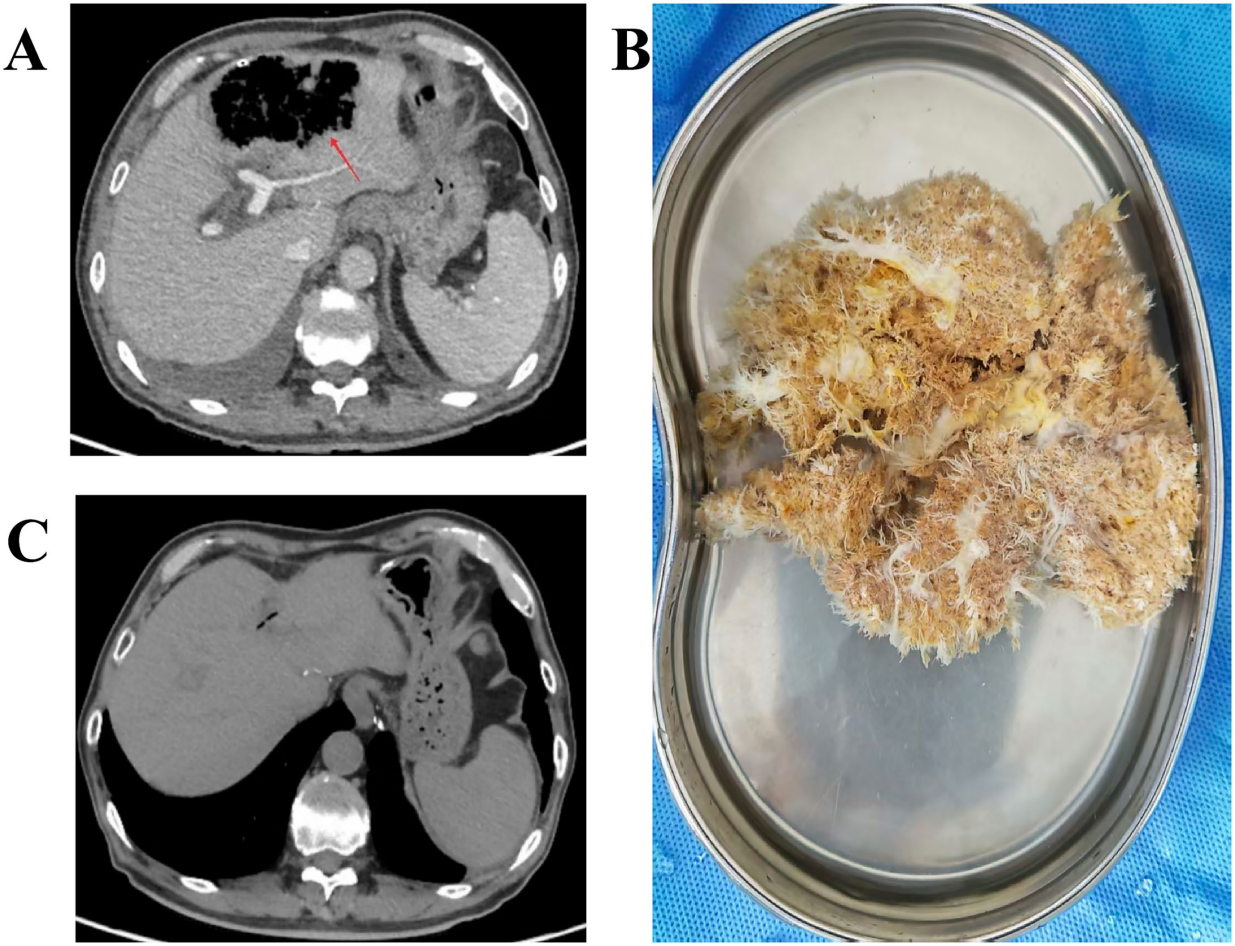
(A) CT showing focal intrahepatic gas accumulation in segments II and III following common hepatic artery embolization (red arrow). (B) Necrotic liver tissue extruded from the laparotomy wound. (C) CT showing regeneration of liver tissue within the previous defect of the left hepatic lobe at 4 months after LPD.
